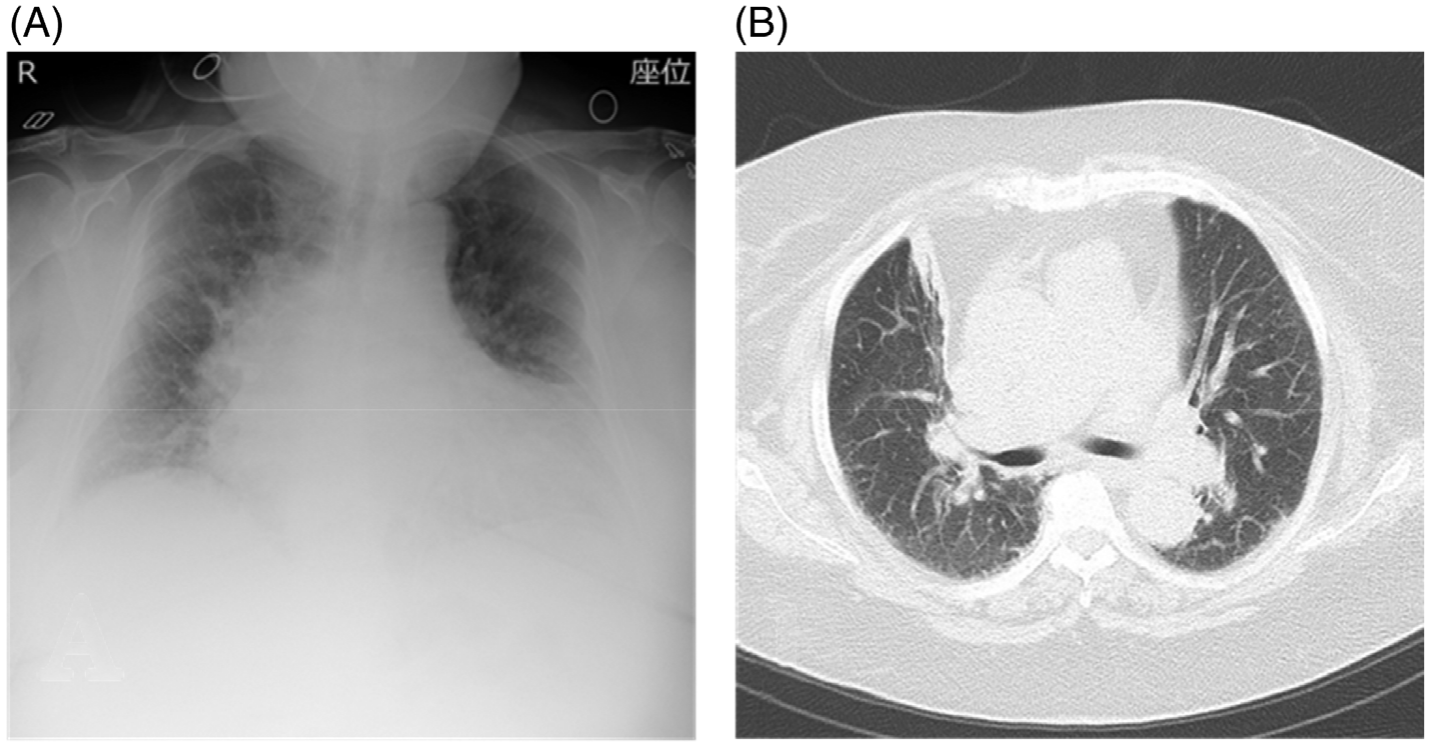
Chest X‐ray and CT of case 4 (A) Chest X‐ray on admission showed an increased cardiothoracic ratio. (B) CT on admission revealed thick subcutaneous fat surrounding the thorax and no obvious infiltration in the pulmonary fields
